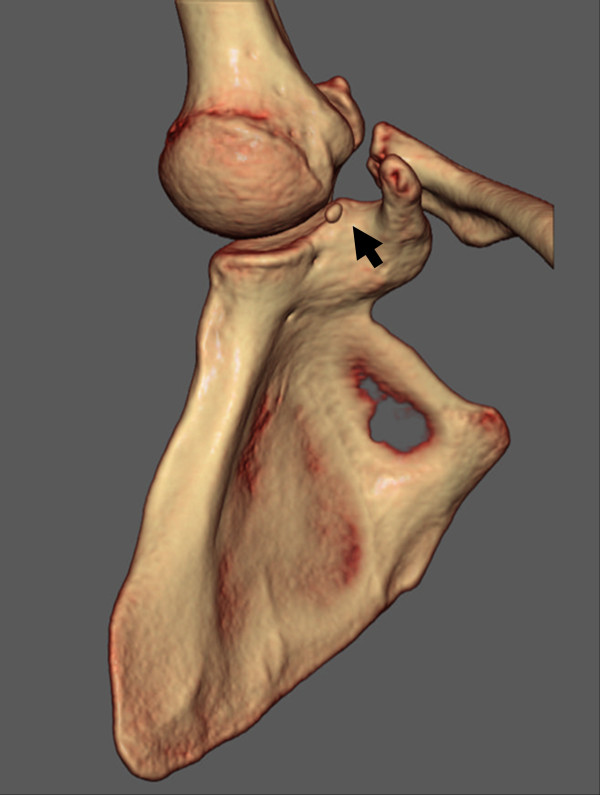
A three-dimensional-computed tomography (3D-CT) image shows a small bony fragment located between the humeral head and the glenoid of the scapula (arrow).
